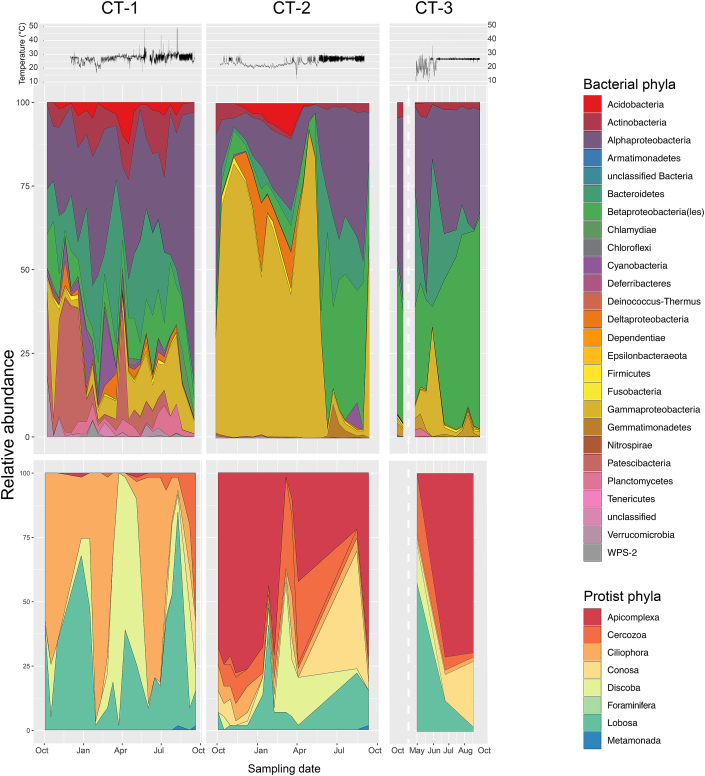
Temporal dynamics of bacterial and protists diversity over a one year sampling period. Changes in relative abundance of bacteria and protists at the phylum level for cooling towers CT-1, CT-2, and CT-3 reveals temporal dynamics of community structure despite relatively constant environmental conditions. The average daily temperature data in the water basin is shown in the uppermost panel; lack of data for CT-3 is indicated by a white dashed line.
